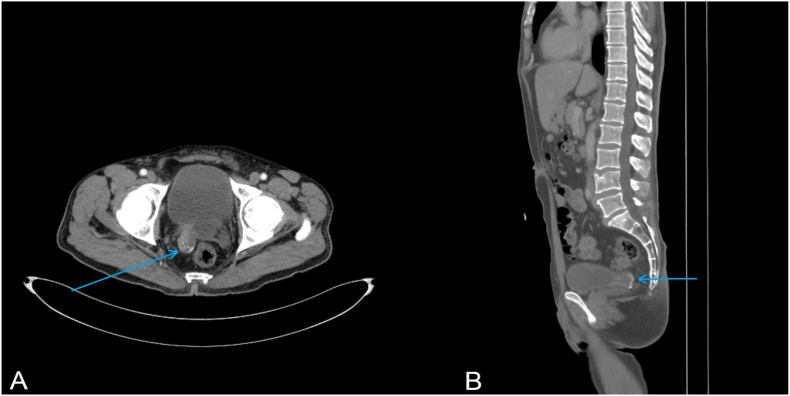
A and B CT scan revealing a small bladder diverticulum (26mm × 37mm) on the right posterior wall of the bladder.
